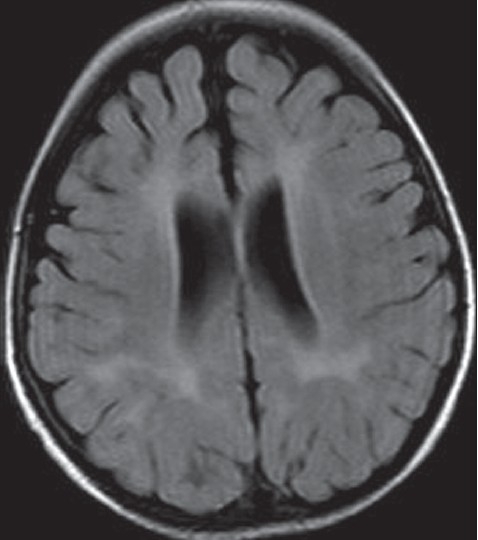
MRI (FLAIR) in a 20 months old girl with phenylketonuria. Note the periventricular hyperintensities
